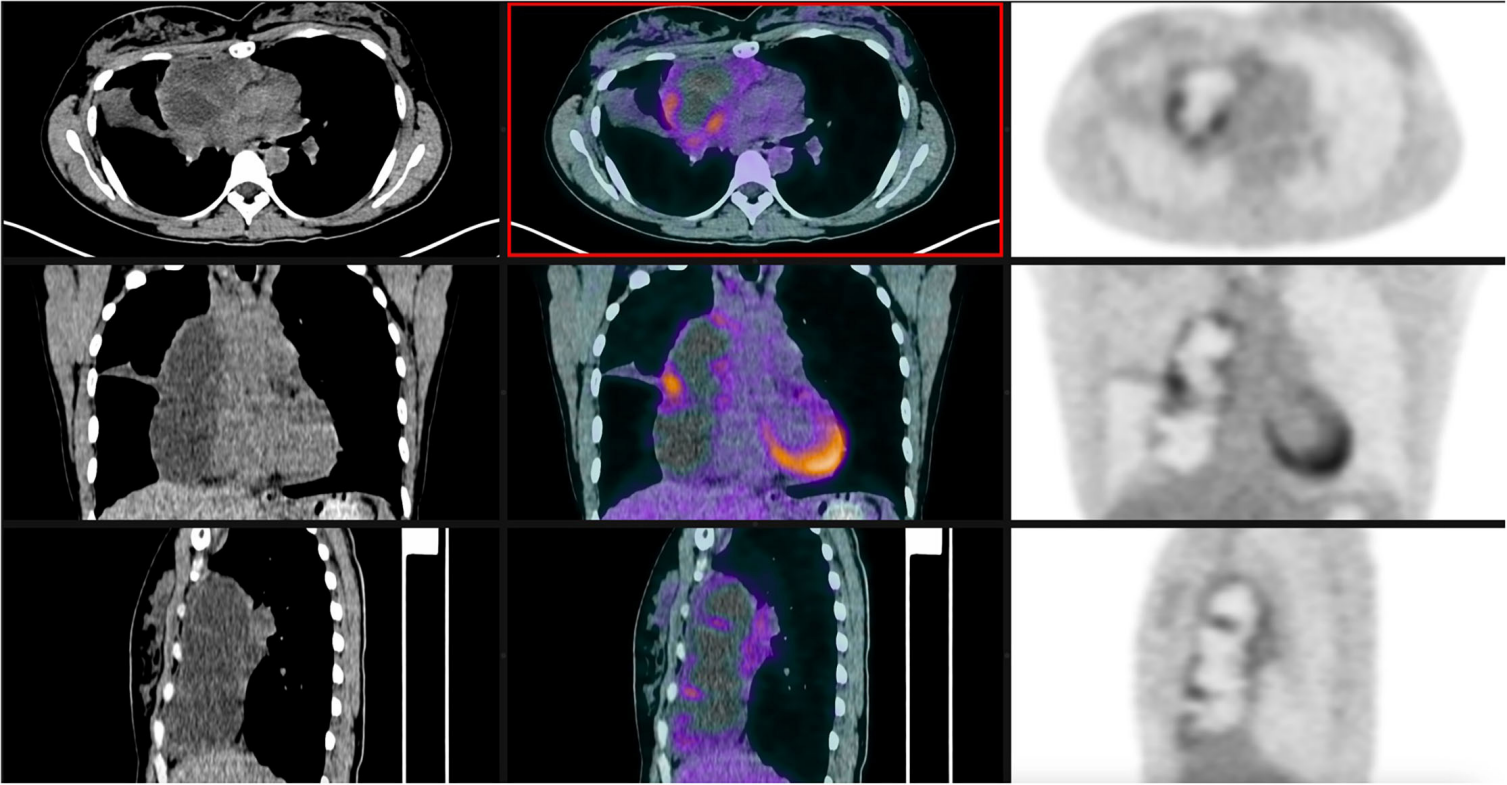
^18^FDG PET-CT scan showing hypermetabolic activity at the level of the mass.
